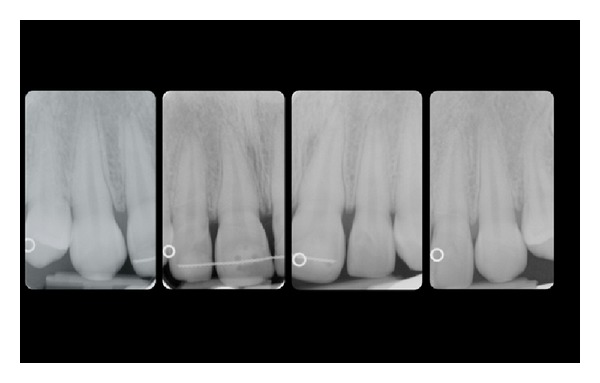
Periapical X-ray of the upper anterior teeth to plan sub-marginal incision (see test for explanation).
